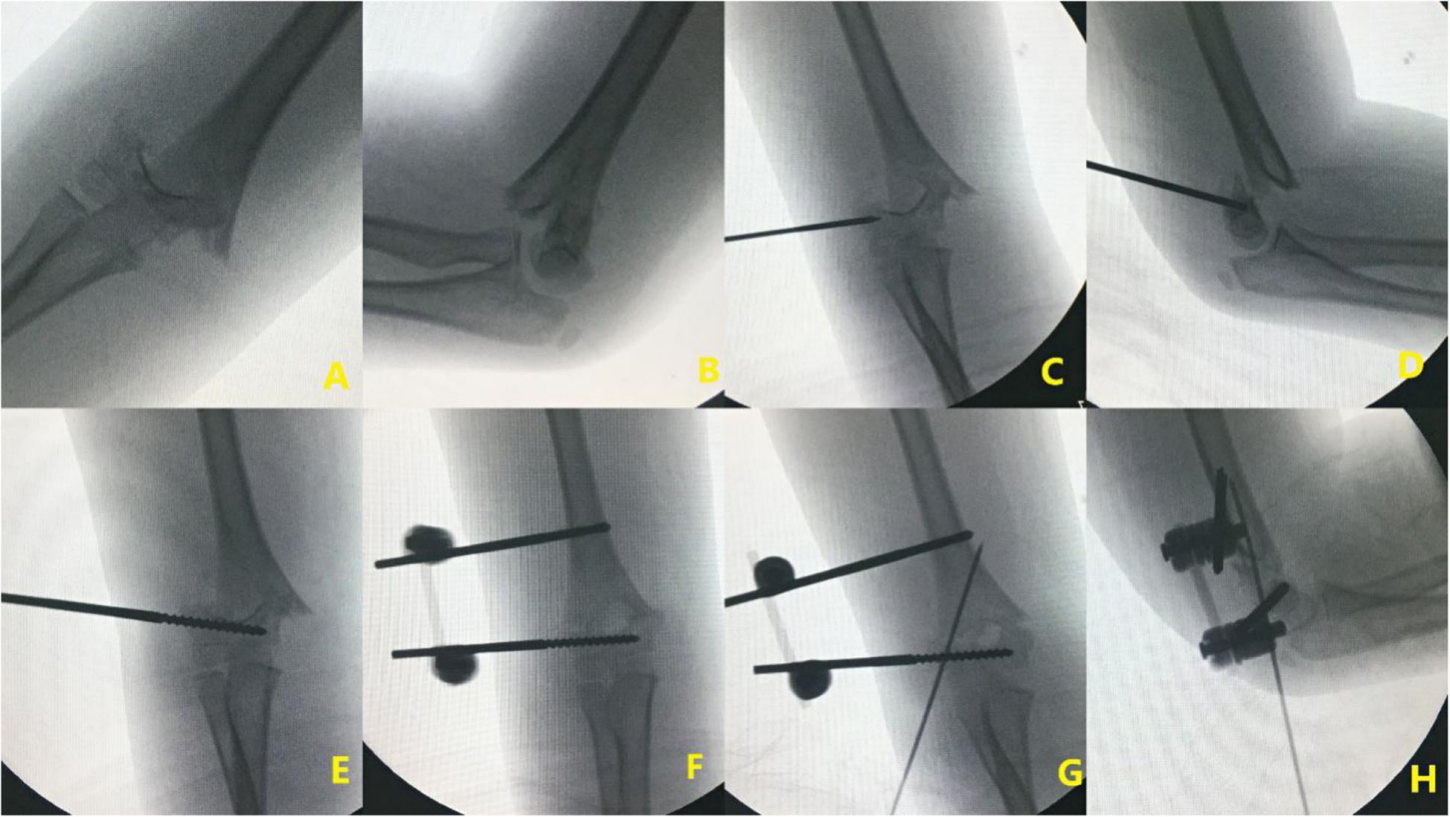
Preoperative X-ray radiographs (A, B); location and placement of the distal Schanz screw (C–E); placement of proximal Schanz screw (F); anteroposterior and lateral X-ray radiographs after anti-rotating Kirschner wire placement (G, H)
